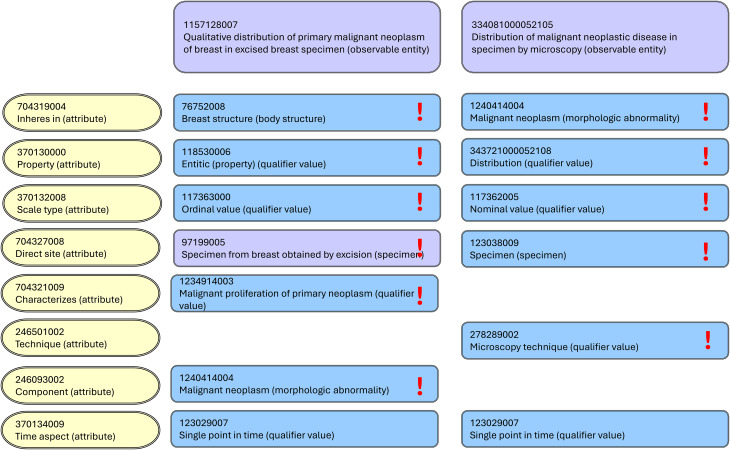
Example of a non-transformable concept pair. The two concepts are very differently modelled and not transformable via the SNOMED CT concept model, despite “breast” being present elsewhere in the structure
